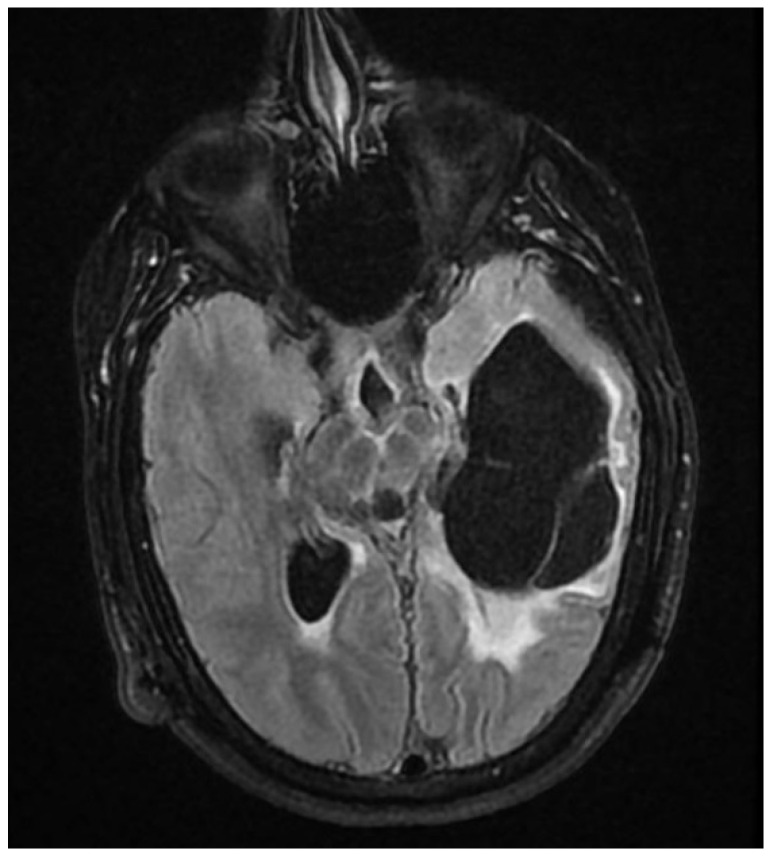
Ax Flair T2 head MRI—septate cystic cavity, with identical signal to CSF, maximum dimensions of 80.3/33/47.1 mm, located temporo-occipital on the left, with neighboring glial changes and presence of the intracavitary tube.
